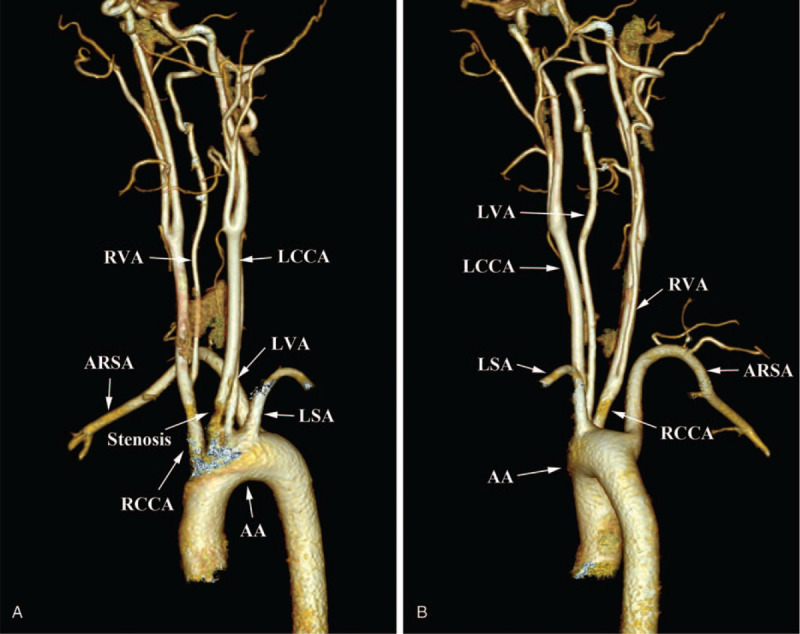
CTA showing the variations of AA branches and the stenosis in LCCA. (A). The 5 branches of the AA (anterior view) and the stenosis in LCCA. (B). The 5 branches of the AA (left lateral view) and the origin of RVA from the RCCA.
